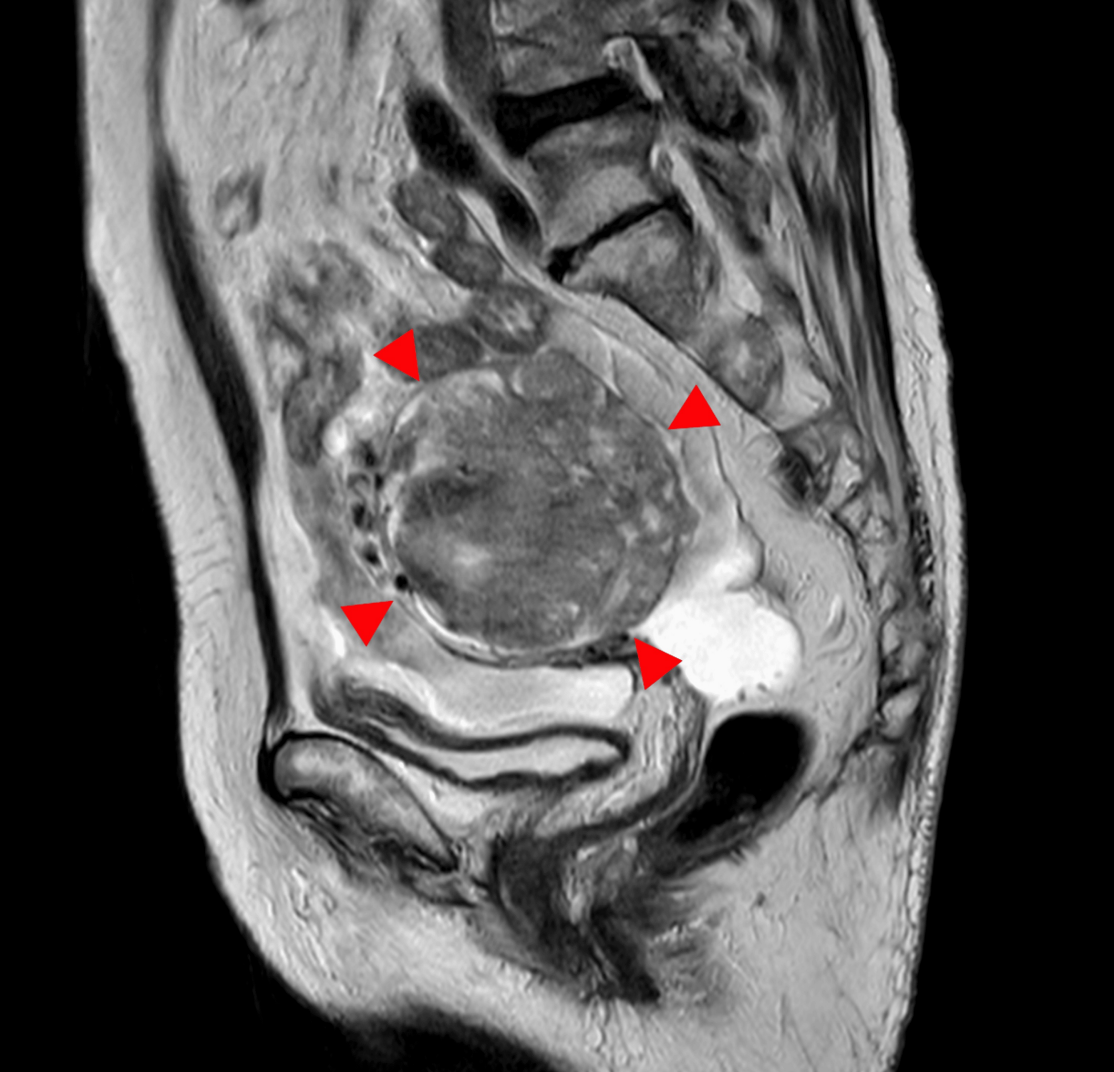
MRI in an 80-year-old woman with ovarian carcinosarcoma of the right ovary The sagittal T2-weighted image demonstrates a well-defined solid mass with heterogeneous signal intensity and partial cystic components (arrowhead). The tumor measures 7.2 cm in diameter.
